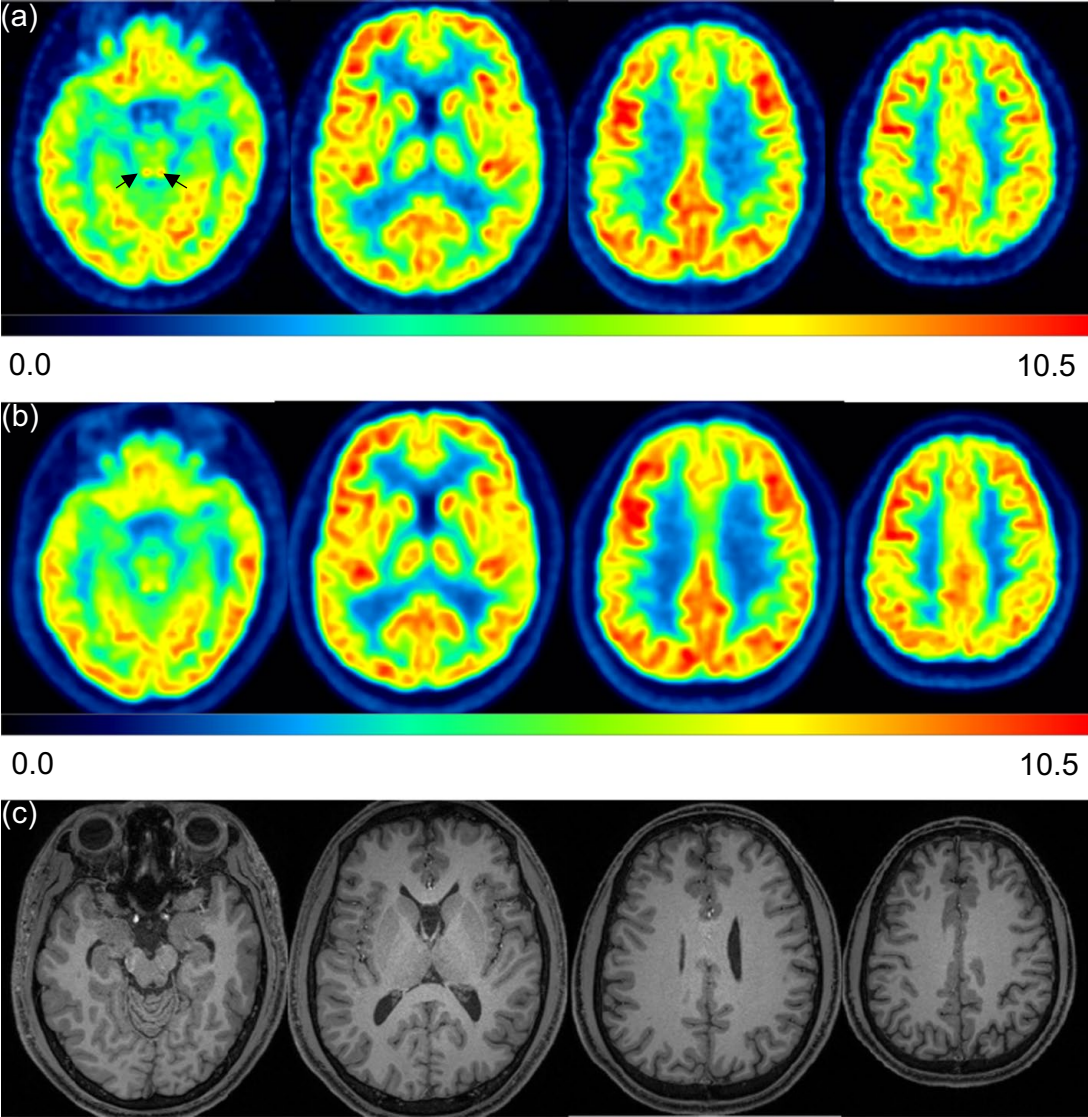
Representative images of FDG of the brain using Vrain (a) and whole-body PET (b). Axial images from the left are at the level of the inferior colliculus (arrows), basal ganglia, centrum semi-ovale, and fronto-parietal area. All PET images were co-registered to T1WI MRI (c). The SUV is indicated by the colour scale
